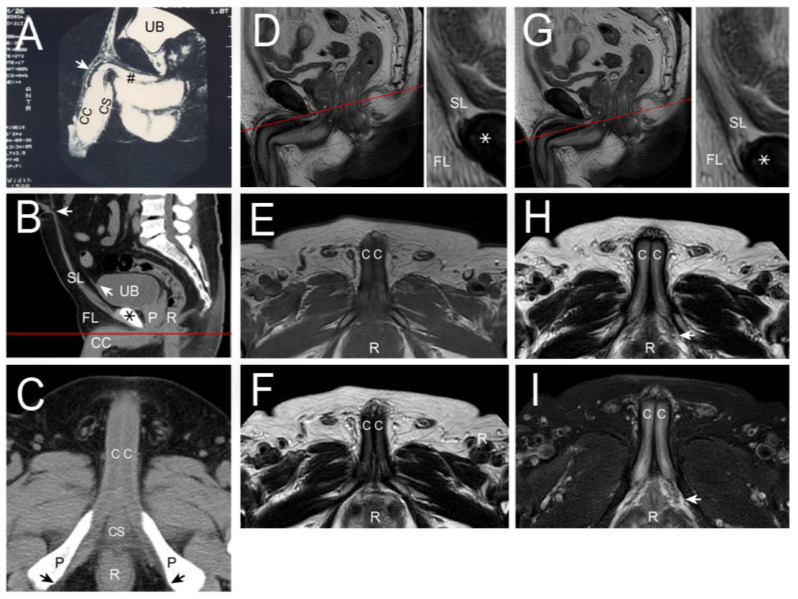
The human penile fibro-vascular assembly obtained from CT and MRI imaging. (A) In the CT imaging, a median sagittal section was made on a 28-year-old man with crural hypoplasia while the penile crus (pound) was petite, whose erection-related veins showed merely the proximal portion (arrow), otherwise masked by sinusoidal opacity. In addition, organoids were demonstrated, for the urinary bladder (UB), corpus cavernosum (CC), and corpus spongiosum (CS). (B) A median sagittal section of the pelvis was conducted to present the symphysis pubis (asterisk), the extension of the urinary bladder (UB) with the median umbilical ligament (oblique arrow), the prostate gland (P), the rectum (R), the suspensory ligament along the linear alba to the umbilicus (left-directed arrow). The section line, red color, was made below the symphysis pubis, resulting in the image of the following one. (C) Given the penis in erection status, it showed the penile corpora cavernosa (CC), (CS), the pubic bones (P), and the rectum (R) ischiocavernosus with cavernosal ligament socketed to the ischial tuberosity (arrow). (D) In MRI imaging, a cross-section was made below the symphysis pubis, red line, for comparison with CT imaging, again demonstrating the suspensory ligament (SL inserted right), fundiform ligament (FL, inserted right) and pubic bone (asterisk). For comparison, imaging was conducted in the T1 mode shown in (E) and T2 mode presented in (F) where the corpora cavernosa (CC) and the rectum (R) are well demonstrated, respectively. Note the right R points the right side. The erection-related veins could be well identified in the T2 mode. (G), however, not intriguing sufficiently. A cross-section was made below the symphysis pubis 2 mm lower, red line, and the mode was conducted in the T2 (H) and 2-FS (I), respectively. Note all the CC, R, FL, and SL mean the same. Overall, the T2 mode was preferred as its enhancing the fibrous tissue of the CC in this study.
